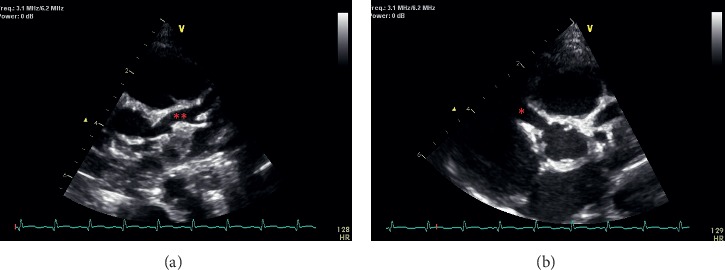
Echocardiogram images from the patient at onset of disease. (a) Parasternal short axis image of the aorta shows a giant fusiform aneurysm of the proximal LAD (^*∗∗*^) and diffuse ectasia of the LMCA. (b) Parasternal short axis image of the aorta shows a giant fusiform aneurysm of the mid RCA (^*∗*^).
